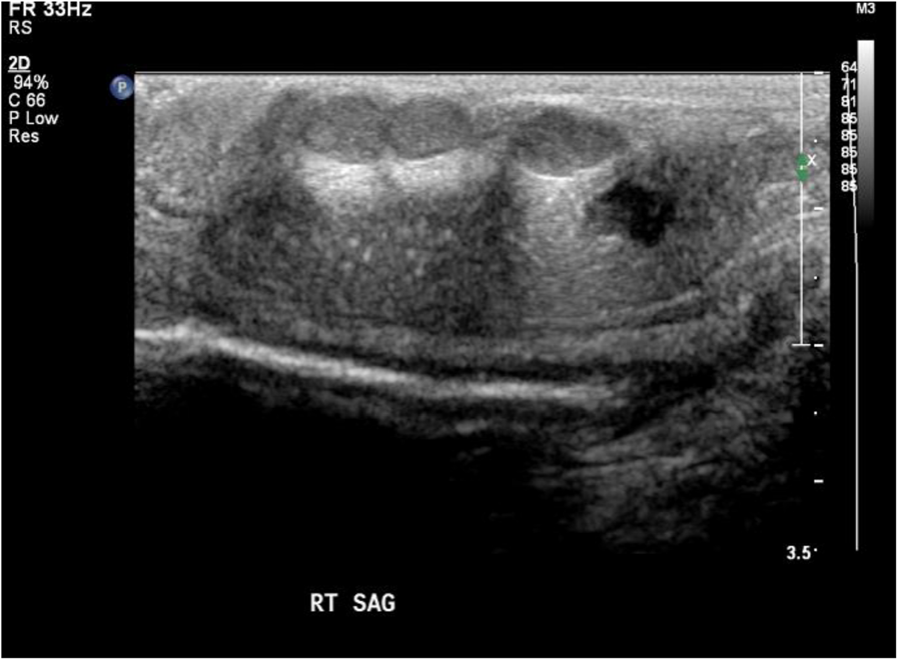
Ultrasound image (longitudinal view) of penile nodules. Ultrasound image (longitudinal view) of penile nodules demonstrates three well-circumscribed, hypoechoic nodules adjacent to the dorsal aspect of the corpus cavernosa
